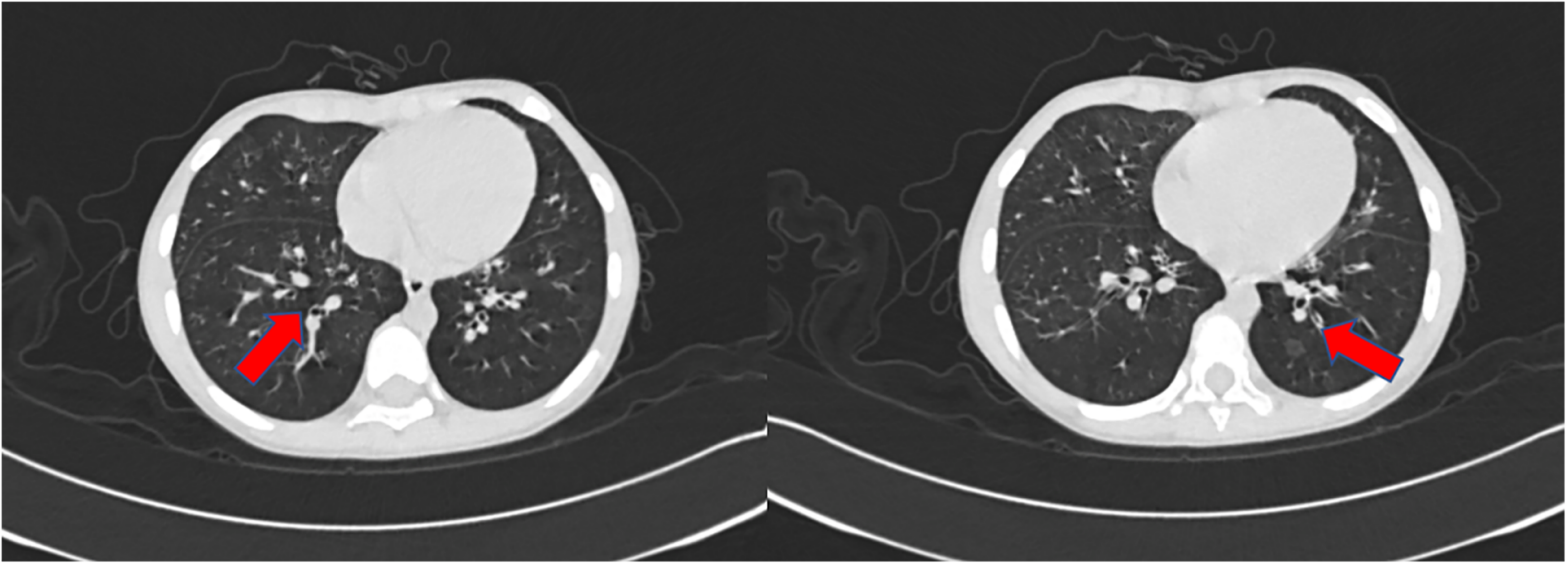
The CT scan of the chest performed 29 days after admission showed a thickening of airway walls.
